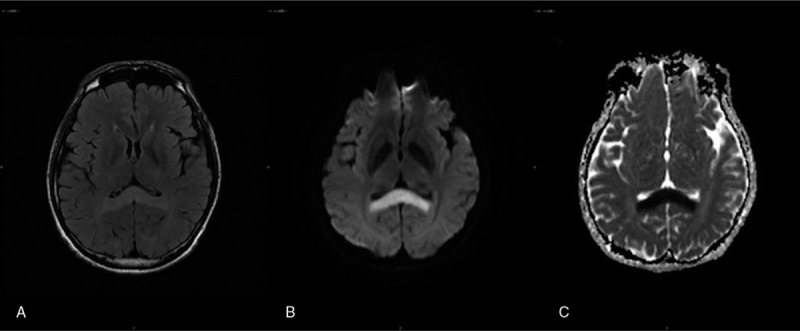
MRI on the day the patient felt difficulty with speech. (A) T2- FLAIR imaging showed hyperintense lesions in the splenium of corpus callosum (white arrow). (B) DWI showed hyperintensity in the corresponding area (white arrow). (C) ADC revealed hypointense lesions in the corresponding area (white arrow). MRI = magnetic resonance imaging; FLAIR = fluid attenuation inversion recovery  ; DWI = diffusion weighted imaging  ; ADC = apparent diffusion coefficient  .
